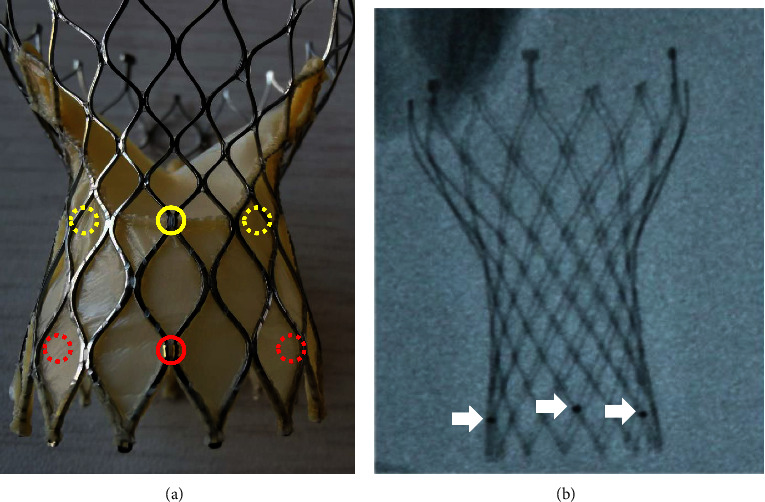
(a) Three markers (red circles) on the inflow of the THV are in alignment with the nadirs of the THV's sinuses (yellow circles). The circles with dotted lines indicate that they are at the back of the THV. (b) The markers can be seen under an X-ray (white arrows). THV = transcatheter heart valve.
